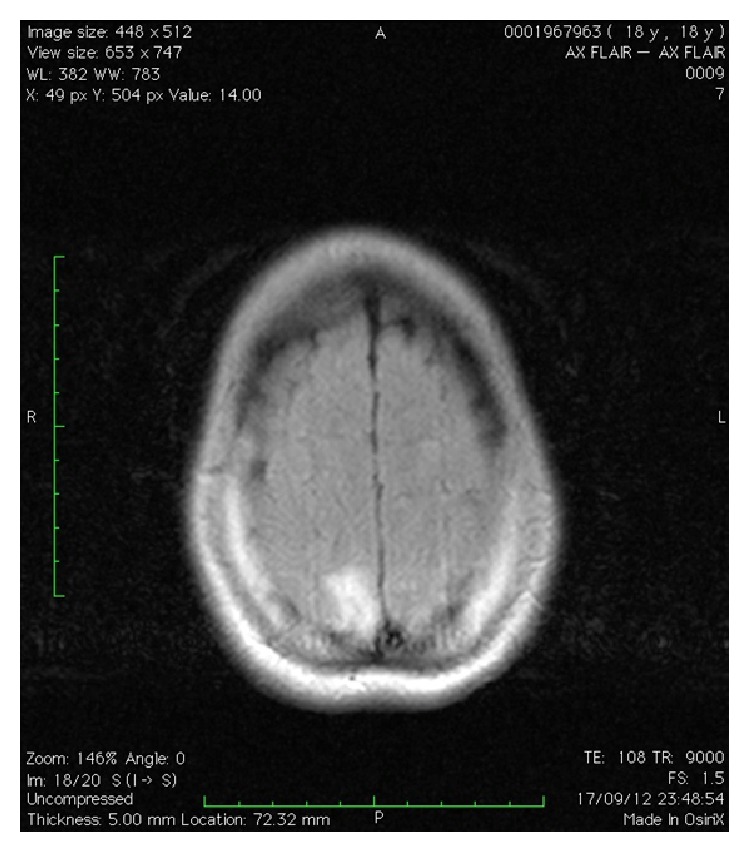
Cranial angioresonance showing cerebral parenchyma with bilateral occipital subcortical areas that present hypersignal in FLAIR and T2, which are associated with a slight expansive effect and do not change after injection of contrast medium, with diffusely compressed cerebral sulci. Alterations compatible with bilateral occipital areas of subcortical vascular disease, which may be present in patients with hypertensive encephalopathy, as observed in cases of preeclampsia known as posterior reversible leukoencephalopathy.
